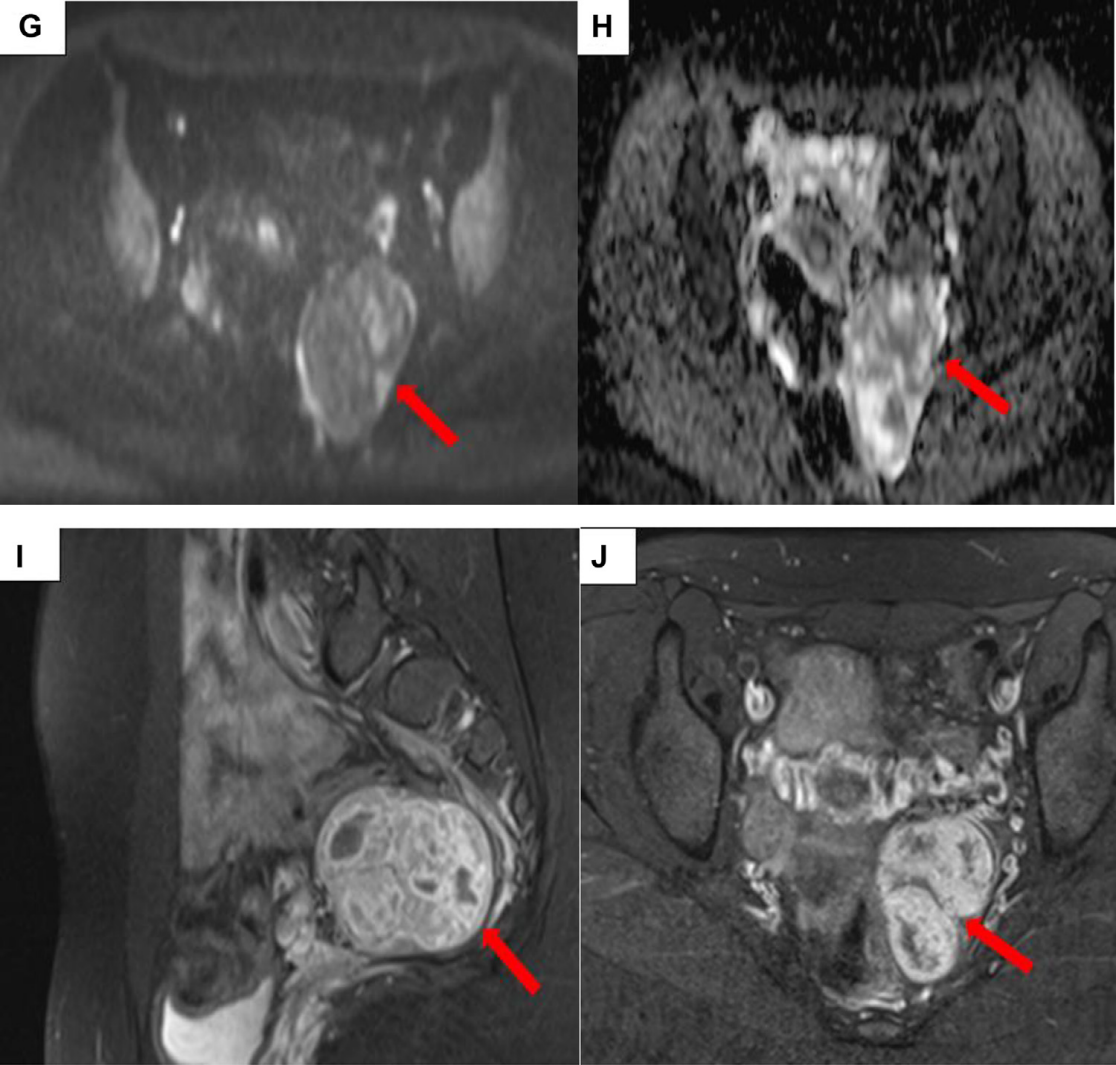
Pelvic MRI: Sagittal T2-weighted (A), coronal T2-weighted (B), axial T2-weighted (C), axial T2-weighted with fat saturation (D), axial T1-weighted (E), axial T1-weighted with fat saturation (F), diffusion-weighted imaging (G), ADC map (H), coronal and axial contrast-enhanced T1-weighted images with fat saturation (I and J). A left lateral uterine mass (red arrow) demonstrating the claw sign with the left ovary (yellow arrow), well-defined, with regular contours and surrounded by a thin capsule. The lesion is heterogeneous, with an intermediate T2-weighted signal, isointense on T1-weighted images, and does not suppress on fat-saturated sequences (confirming the absence of fat components). It exhibits mild hyperintensity on diffusion-weighted imaging with a slightly low ADC, strong enhancement after gadolinium administration, and a minor cystic component with T2 hyperintensity. The right ovary is follicular, with normal size and morphology (green arrow).
